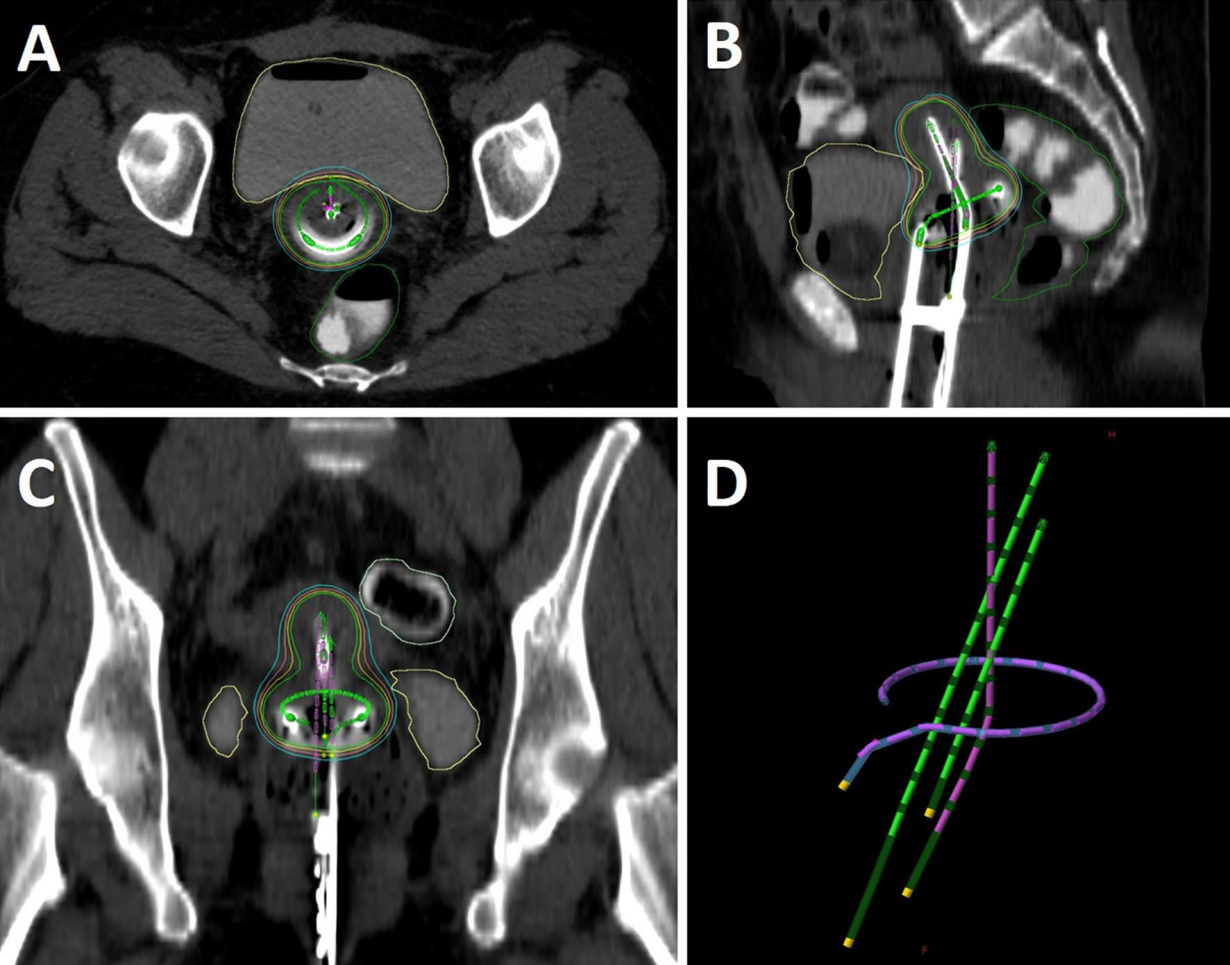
Axial (A), sagittal (B) and coronal (C) views of planning CT with tandem–ring applicator and two additional intracavitary applicators. Three-dimensional reconstruction of the tandem–ring applicator (purple) and additional applicators (green) (D)
